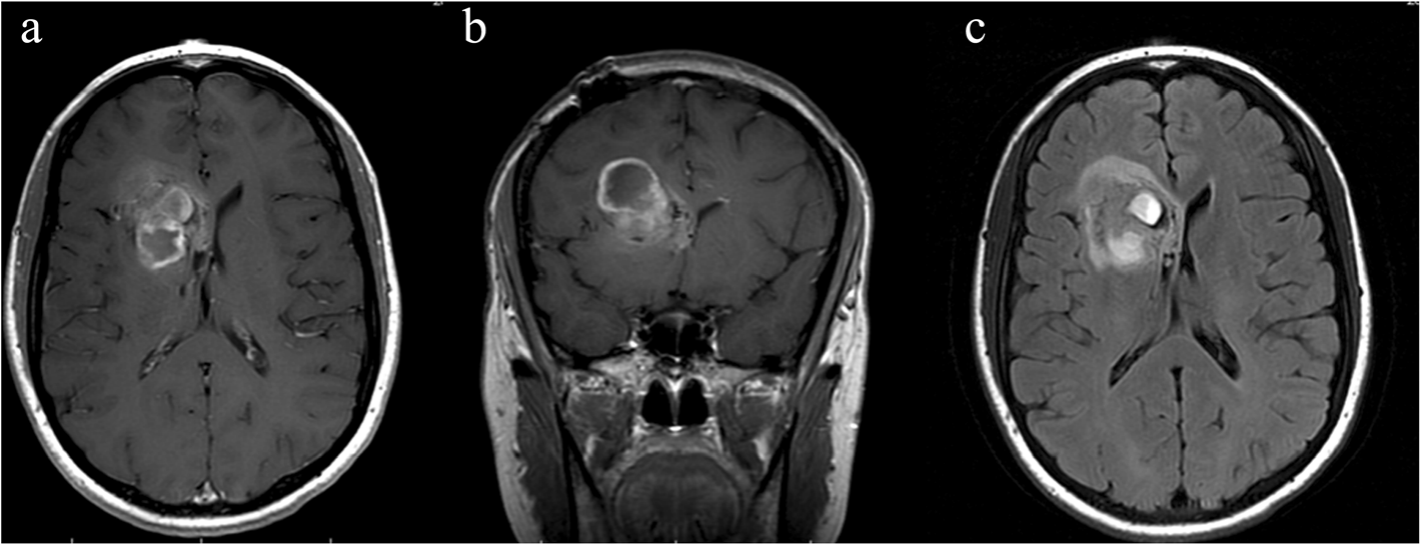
Image example of qualitative invasive phenotype: ependymal extension. 33 year old female patient with right frontal GBM. (a) Axial and (b) coronal post-contrast T1-weighted images demonstrate extension of enhancing tumor into the ependymal region of the frontal horn of the right lateral ventricle. (c) Axial FLAIR image demonstrates non-enhancing tumor as well to extend to the ependymal region.
